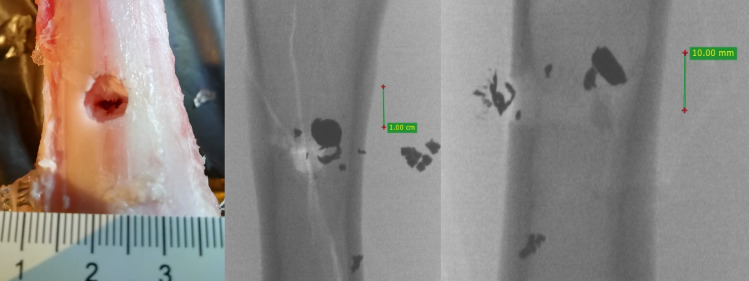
H&N Baracuda Hunter airgun pellet cal. 6.35 mm, on the left:A-P X-ray, in the centre: image of entry damage, on the right: lateral X-ray. Shot to the lateral surface of the femoral shaft.
